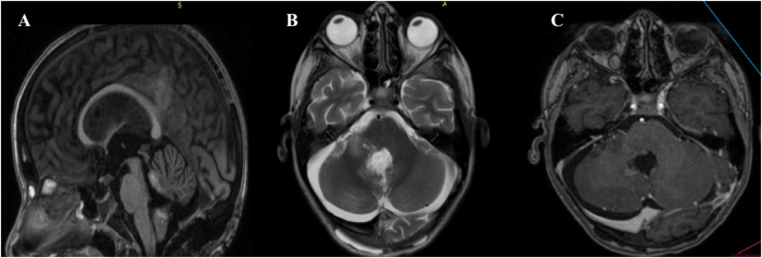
Postoperative MRI after suboccipital craniotomy for a fourth ventricular tumor. (A) Sagittal T1-weighted post-contrast image showing the resection cavity in the fourth ventricle. (B) Axial T2-weighted image demonstrating postoperative changes and a small residual lesion in the cerebellopontine angle. (C) Axial T1-weighted post-contrast image confirming residual enhancing tissue adjacent to critical neurovascular structures.
